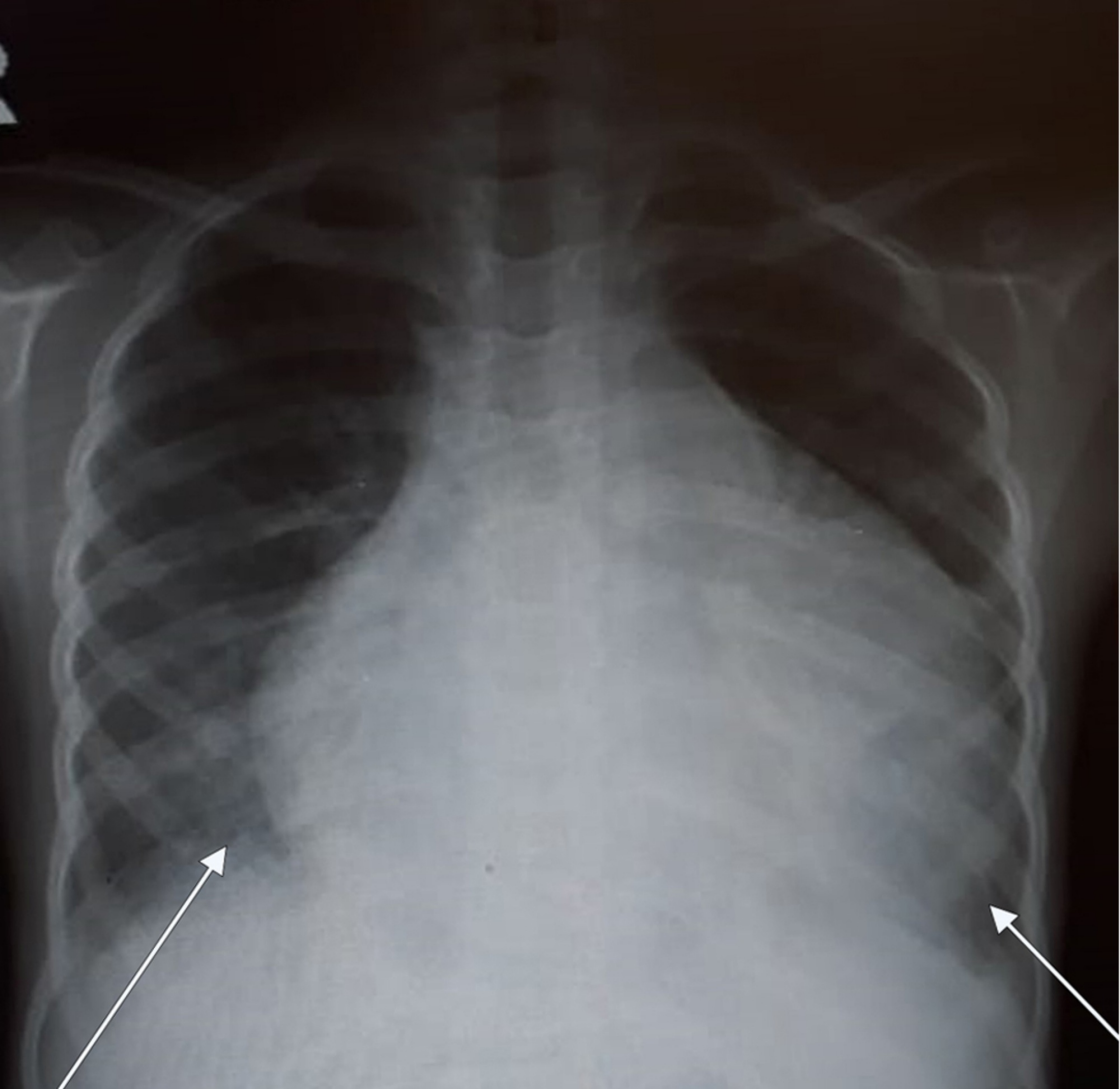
Chest radiograph showing enlarged cardiac silhouette
